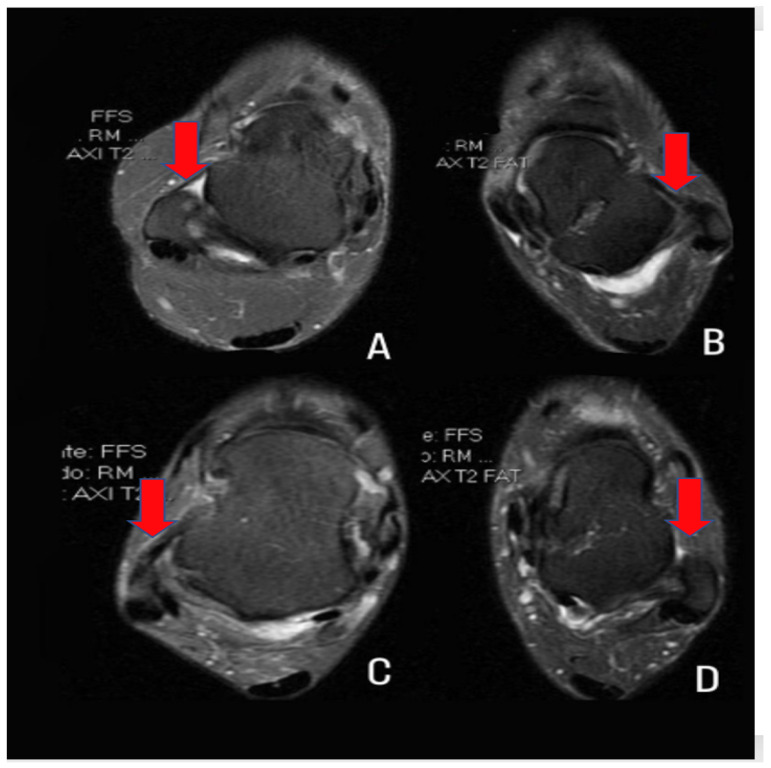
MRI of the ankle in a T2-weighted axial section at the height where the talus is most visible for ATFL analysis. (A): normal ligament (rectilinear and homogeneous); (B): abnormal ligament due to altered signal; (C): abnormal ligament due to altered contours; and (D): absent ligament.
